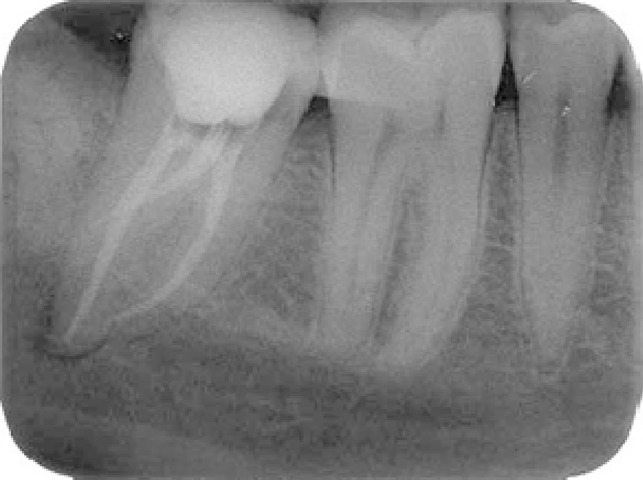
Postoperative radiographs. Three separated root canals were filled with gutta-percha and sealer
